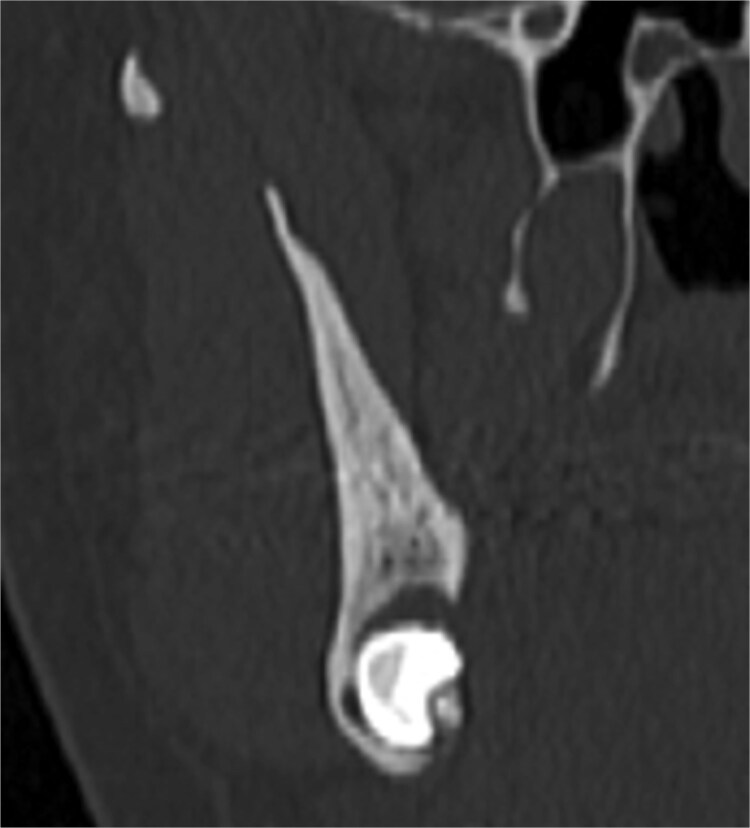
Four months post-operative orthopantomography.
